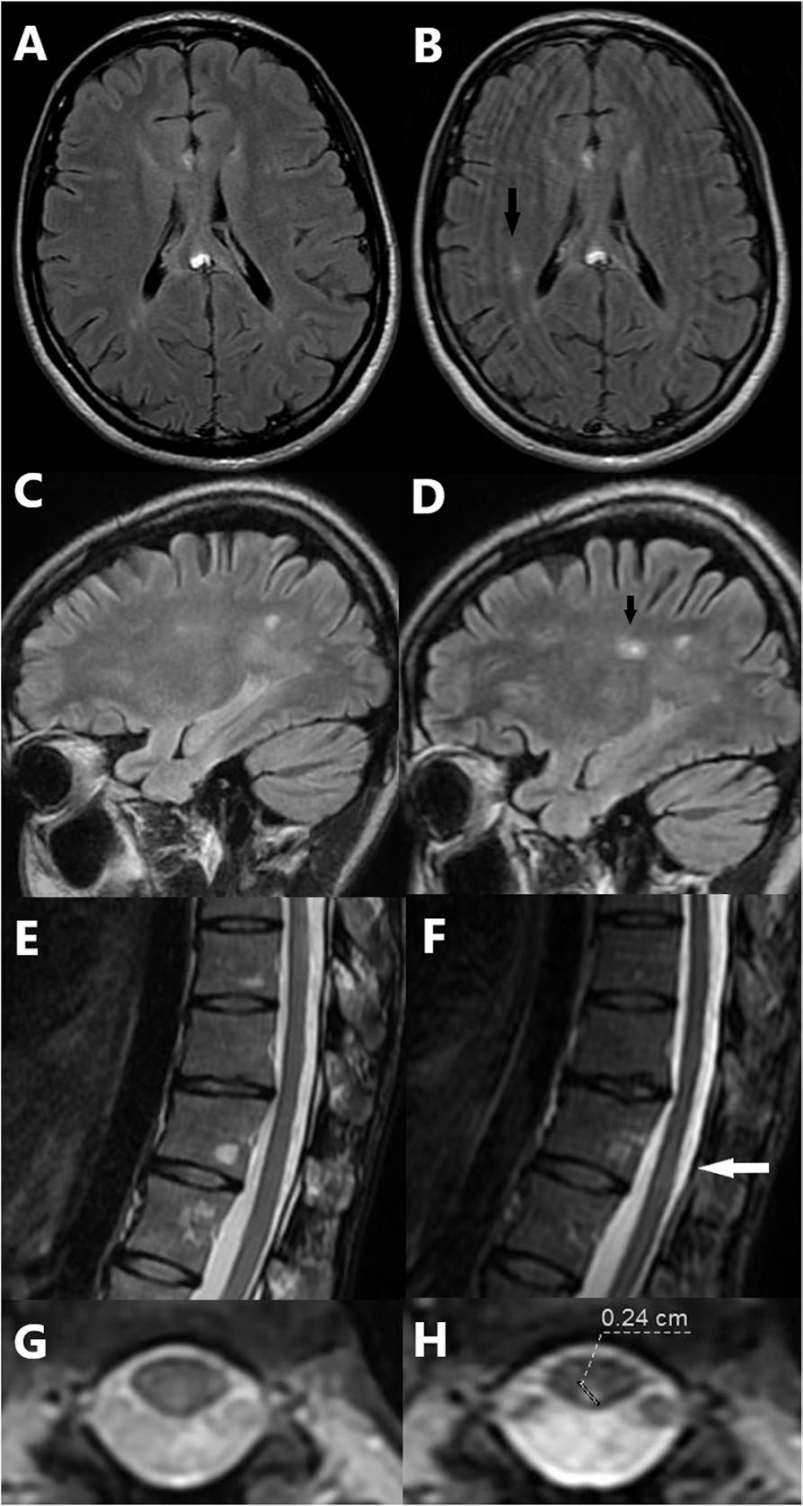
Brain and thoracic spine MRI scans: first admission (left) and second relapse (right). Axial FLAIR and sagittal FLAIR CUBE scans of the brain (B, D) as well as sagittal and axial T2 scans of the lower thoracic spinal region (F, H) taken in 2018 present novel lesions (black arrows, white arrow) compared to scans of the same quality and level from 2013 (A, C, E, G)
